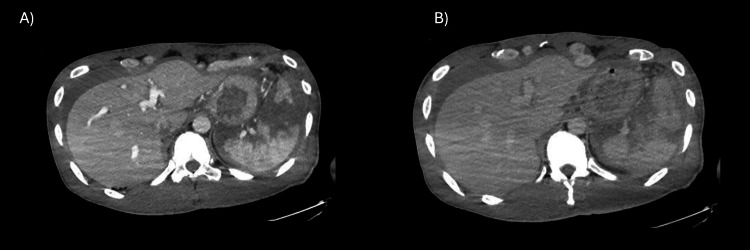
Enhanced whole-body CT axial view (A) arterial phase and (B) portal-venous phase Neither extravascular leakage images nor pseudoaneurysms are seen. CT: computed tomography
